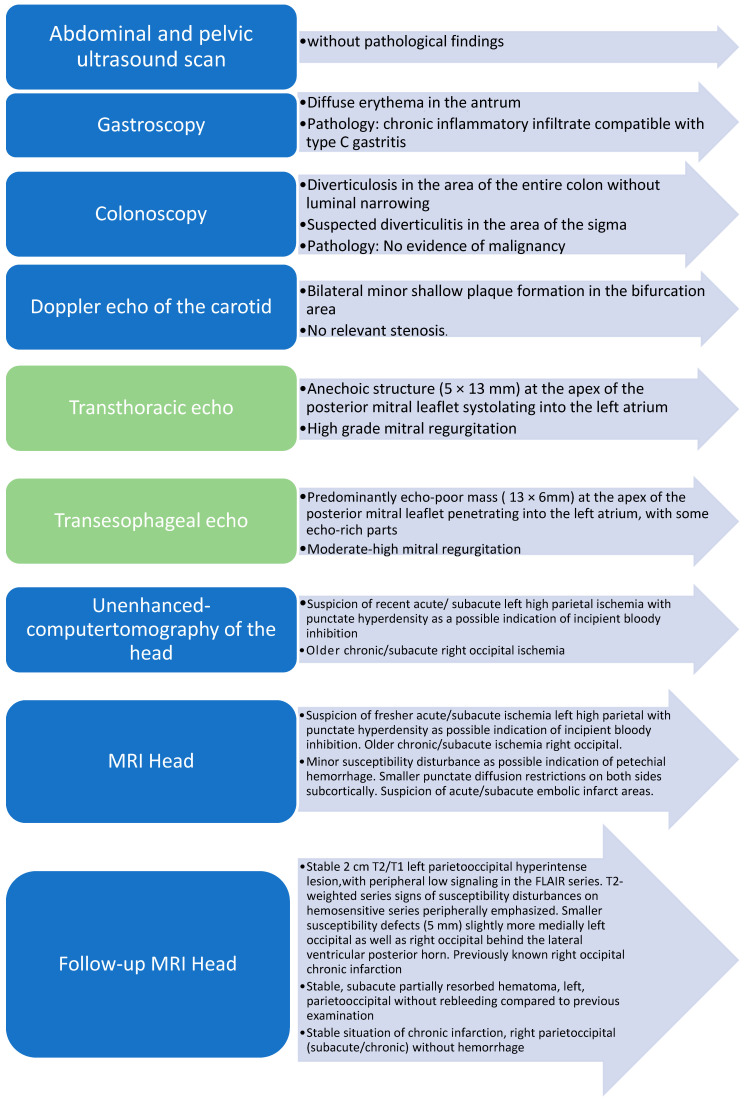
Patient’s diagnostics during hospitalization; green indicating: signs of infective endocarditis. (echo:_echocardiography, MRI: magnetic resonance imaging).
